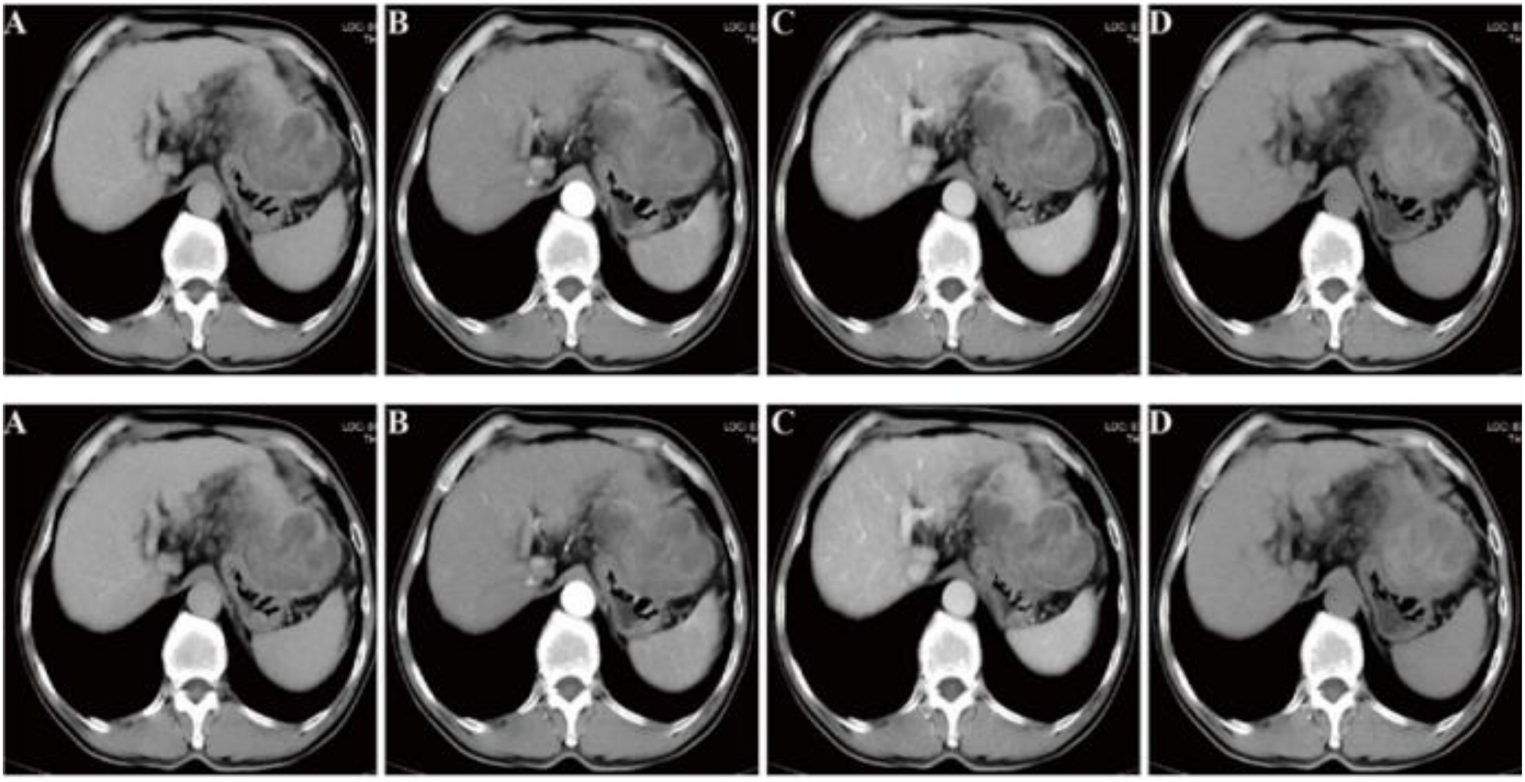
CT findings: (A) Plain CT scan reveals a sinuous appearance of the hepatic margin and an unusual mass in the hepatogastric region. (B) The tumor receives blood supply from the left hepatic artery, exhibiting flocculent increase during the arterial phase. (C, D) The tumor exhibits heterogeneous enhancement during the venous phase and delayed phase.
